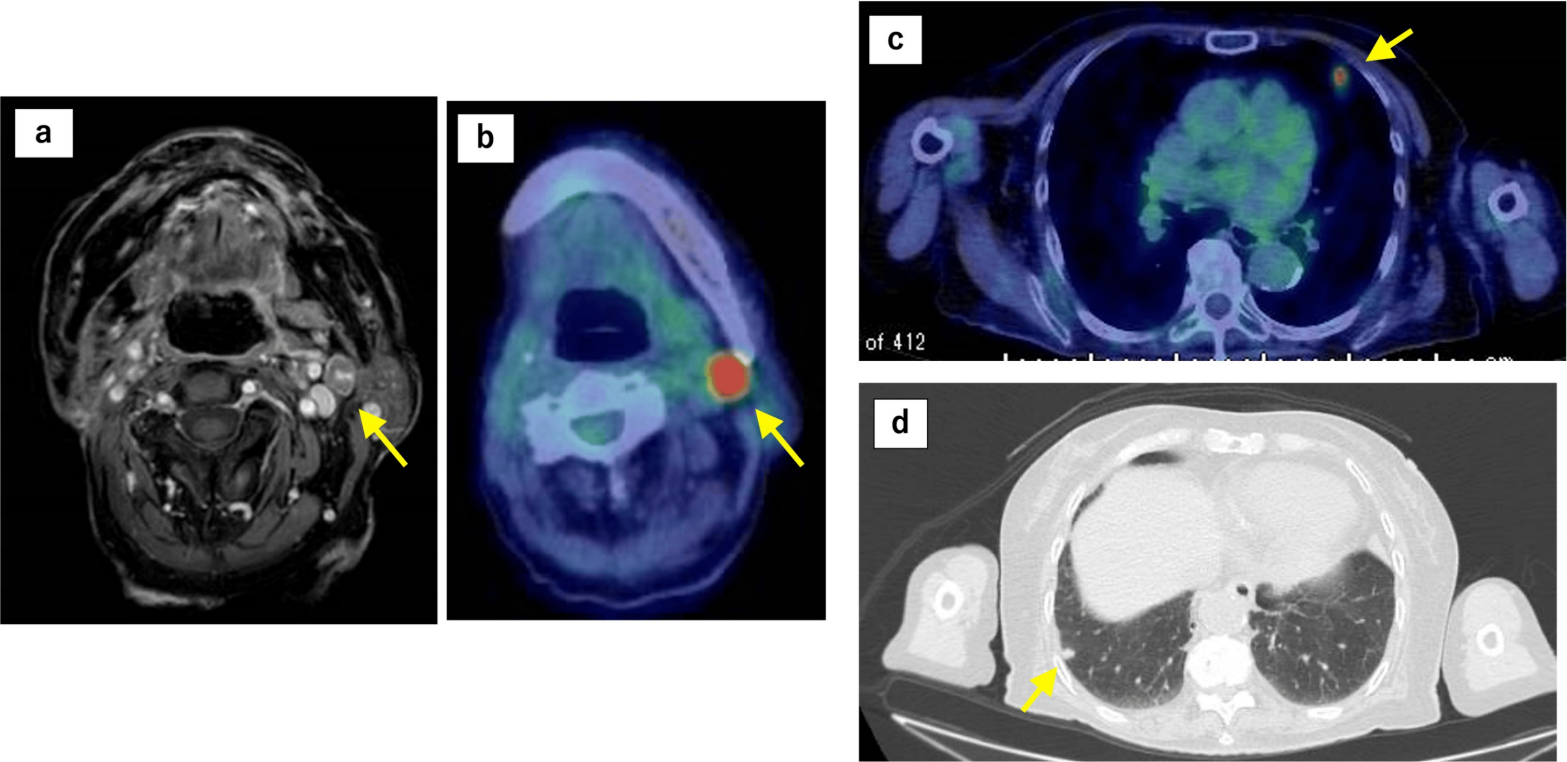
Baseline imaging after initial surgery prior to first SBRT PET-CT images. (a and b) Recurrence of left cervical lymph nodes, (c) metastatic lung tumor in left lung S5, and (d) right lung S9 SBRT: stereotactic body radiotherapy; PET-CT: positron emission tomography-computed tomography
